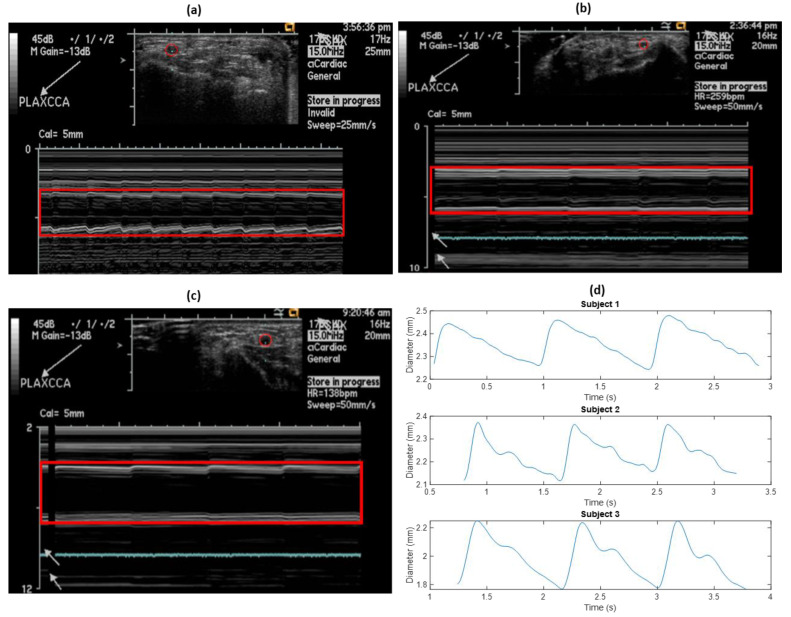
(a–c) Radial artery diameter changes measured using ultrasound for subjects 1, 2 and 3, respectively. The artery is outlined by a red circle and the corresponding M-mode diameter wave is outlined using a red rectangle. (d) Extracted diameter waveforms for the subjects from ultrasound measurements.
